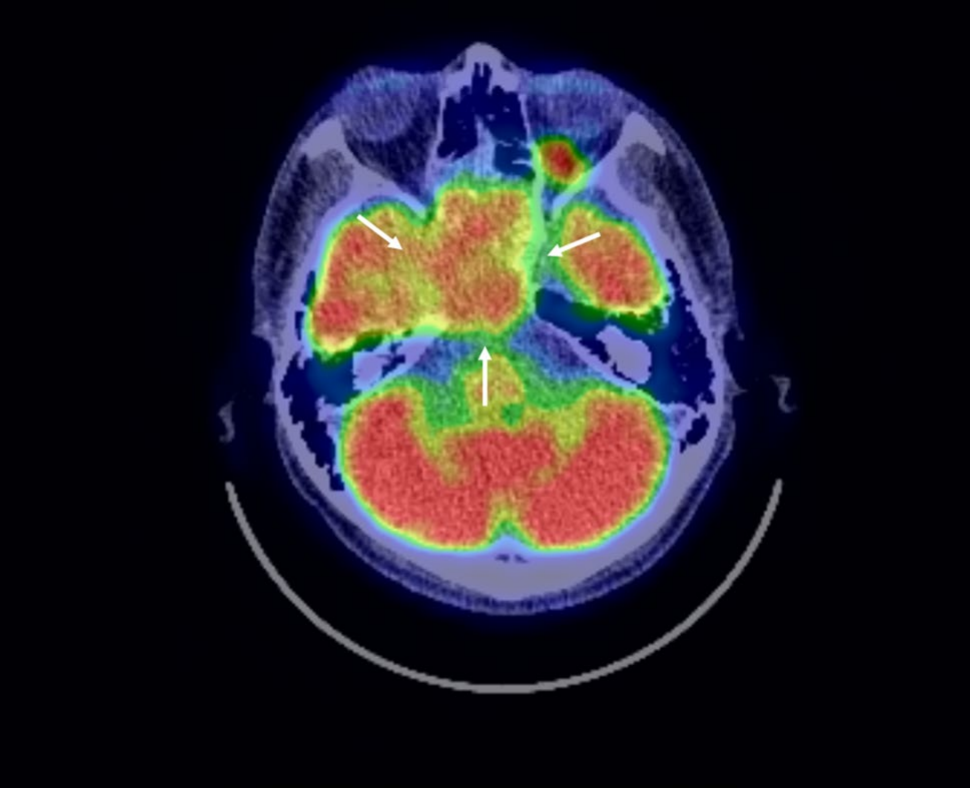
Positron emission tomography-CT (PET-CT) showed uptake of 18F-2-fluoro-2-deoxy-d-glucose in the sphenoid sinus (maximum standardized uptake value of 15.83) (white arrow)
